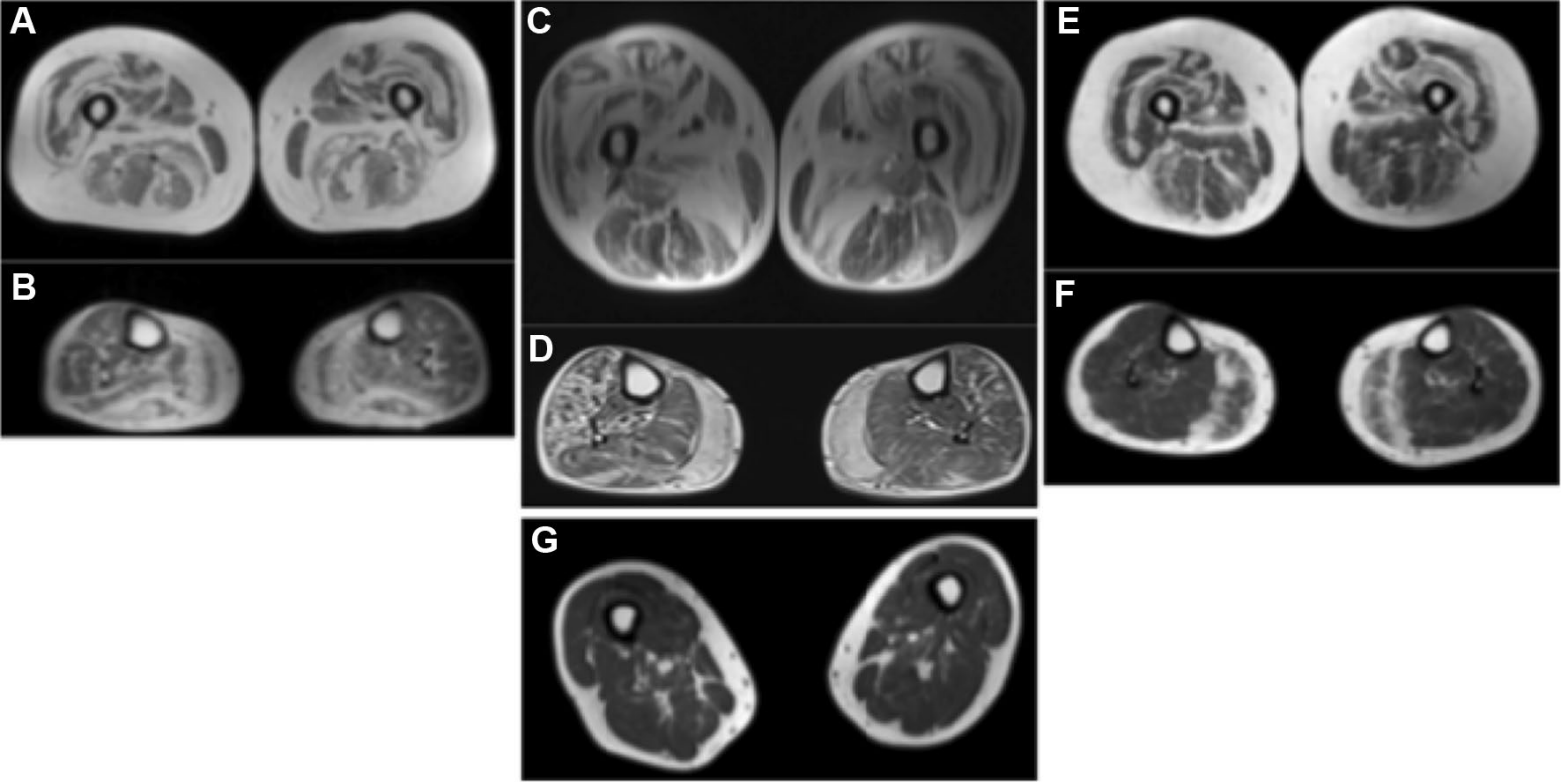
Lower limb magnetic resonance imaging shows characteristic fatty degeneration in the center of the rectus femoris and a layered, "tigroid" pattern in the vastus lateralis of patients II-1 (a), II-2 (c), II-3 (e), while thigh MRI was normal in the unaffected sibling II-4 (g) at 60 years of age. There is severe fatty replacement of the gastrocnemius medialis in all patients (b, d, f)
